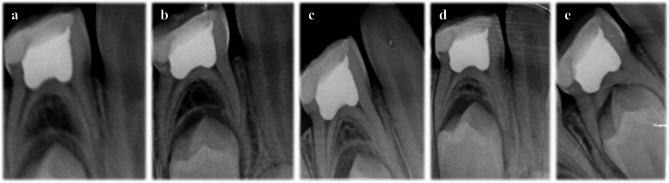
No pathological radiographic finding of the primary mandibular right first molar with Hyaluronic acid until its physiological exfoliation time. (a) 1st-month, (b) 3rd-month, (c) 6th-month, (d) 12th-month, (e) 26th-month
